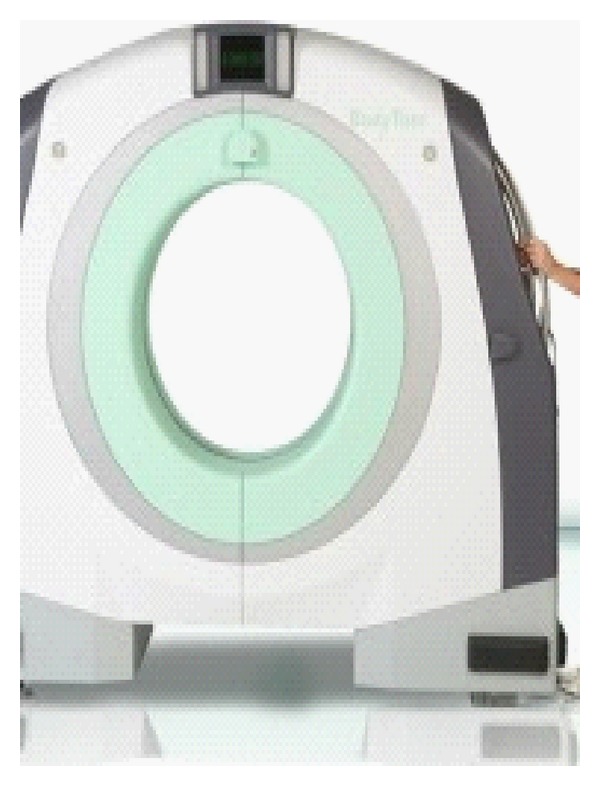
The “Bodytom” portable full body CT scanner. Courtesy of Neurologica.
